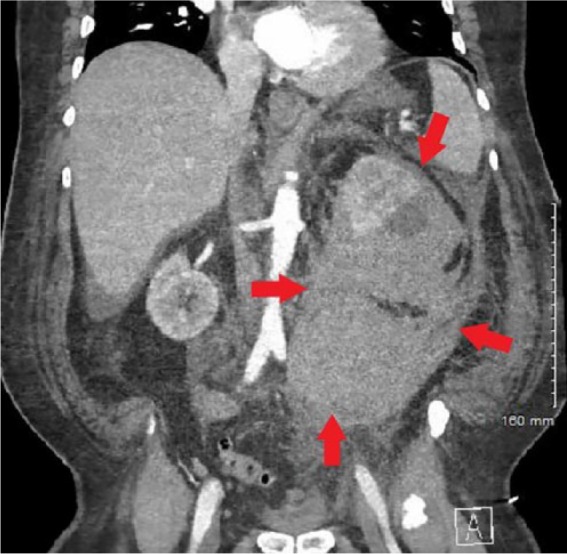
Computed tomography angiography scan of abdomen (coronal) showing retroperitoneal hematoma in the left suprarenal fossa and retroperitoneum extending into the left hemipelvis.
